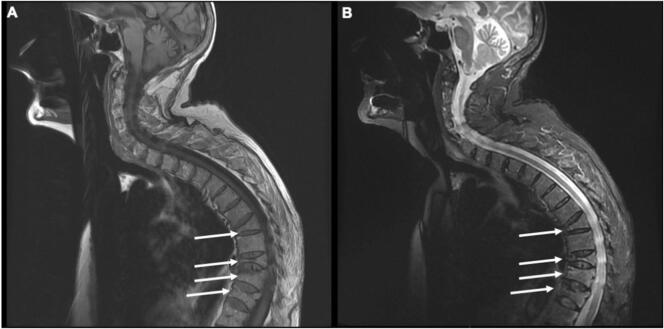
Multiple vertebral fractures(arrows) with osteoporotic appearance without myeloma defining criteria in T1-weighted (A) and T2 STIR-weighted sequences (B) in a patient with smoldering myeloma.
